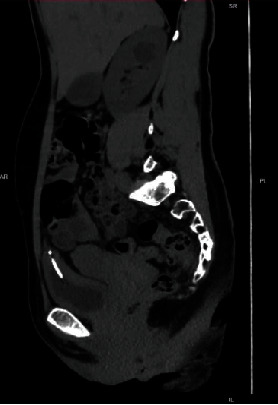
Sagittal CT: IUCD implanted within the dome of the urinary bladder.
